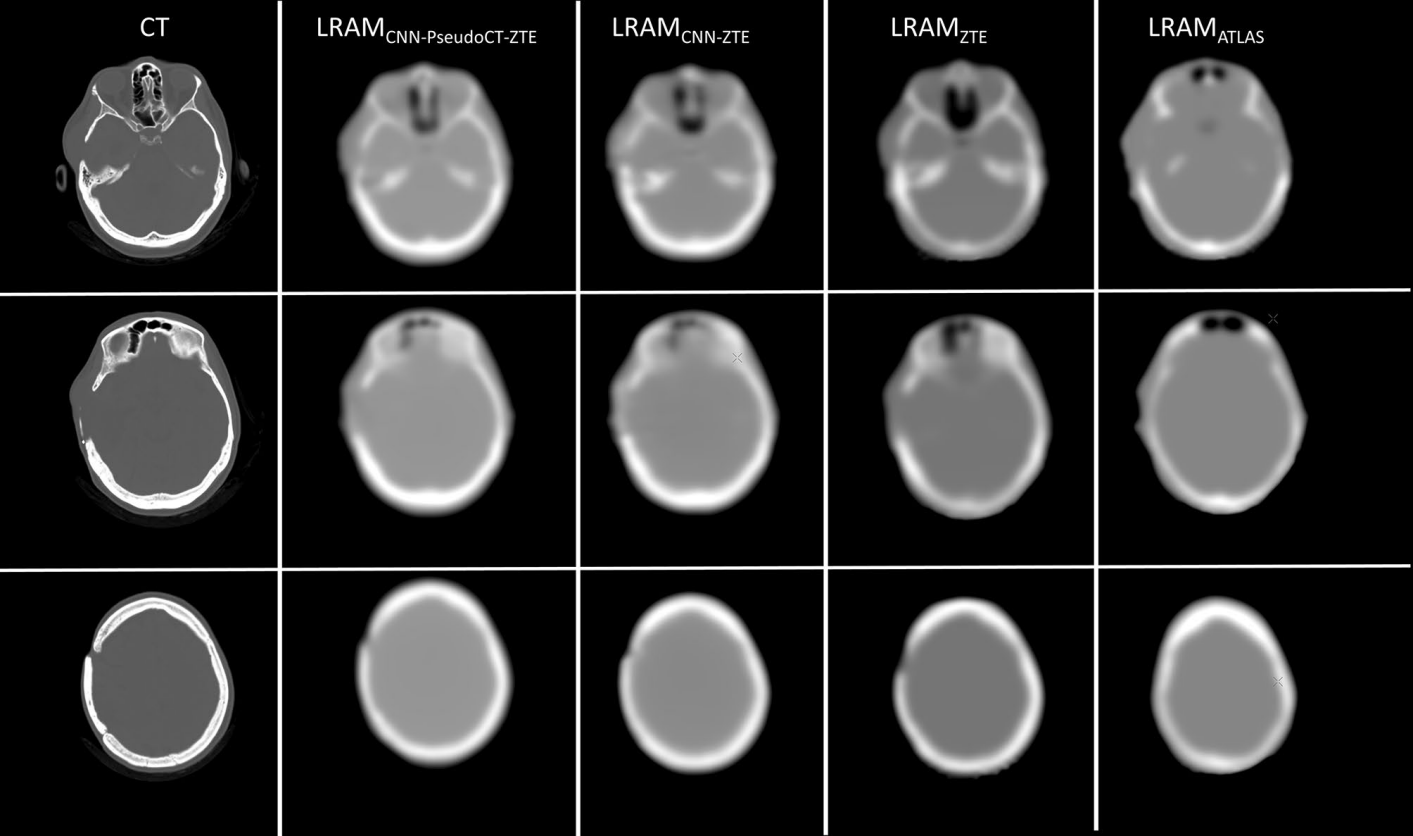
Three representative sections of a METH patient: original CT (left) and corresponding LRAMs, LRAM_CNN-pseudoCT-ZTE_, LRAM_CNN-ZTE_, LRAM_ZTE_, LRAM_ATLAS_
